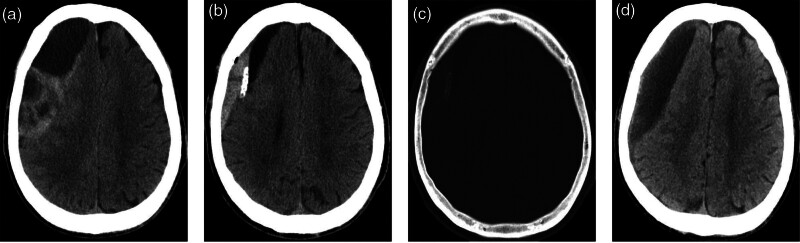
Axial CT scans of case 2. (A) Preoperative image; (B) acute right-sided subdural hemorrhage at 19 h postoperatively; (C) hemorrhagic lesion encasing the drainage tube; (D) increased subdural effusion at 30 days postoperatively. CT = computed tomography.
